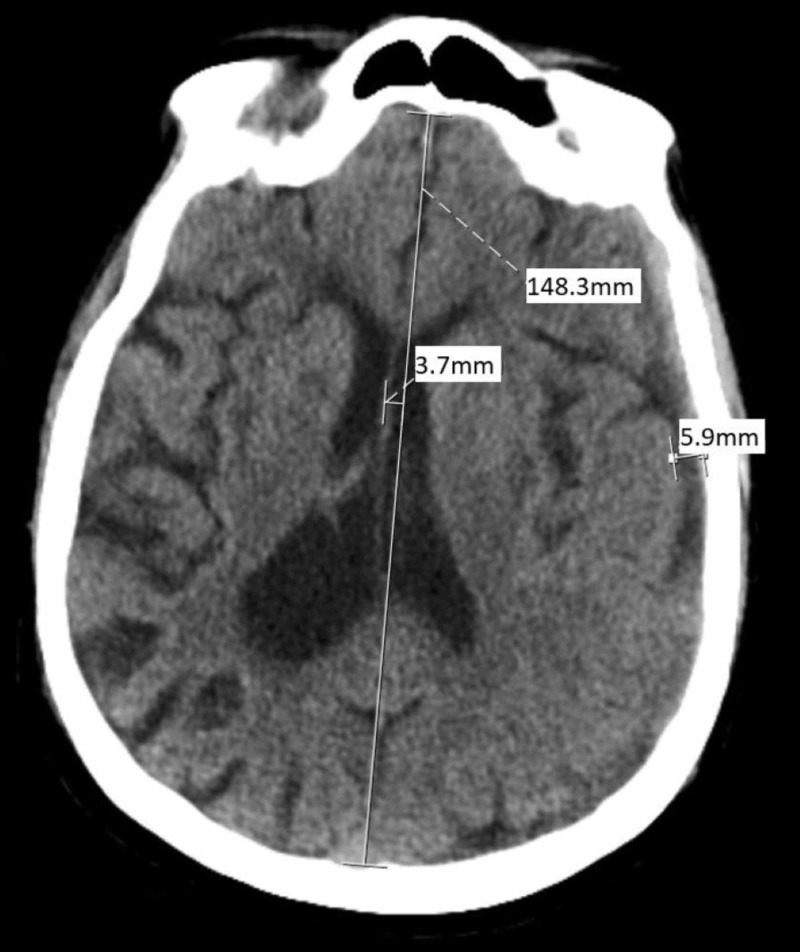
POD 3 CT examination completed 11 hours after 2 mg infusion of tPA demonstrates continued hematomal drainage with only 3.7 mm left to right midline shift and maximal SDH depth reduced to 5.9 mm. POD: postoperative day; CT: computed tomography; tPA: alteplase; SDH: subdural hematoma
